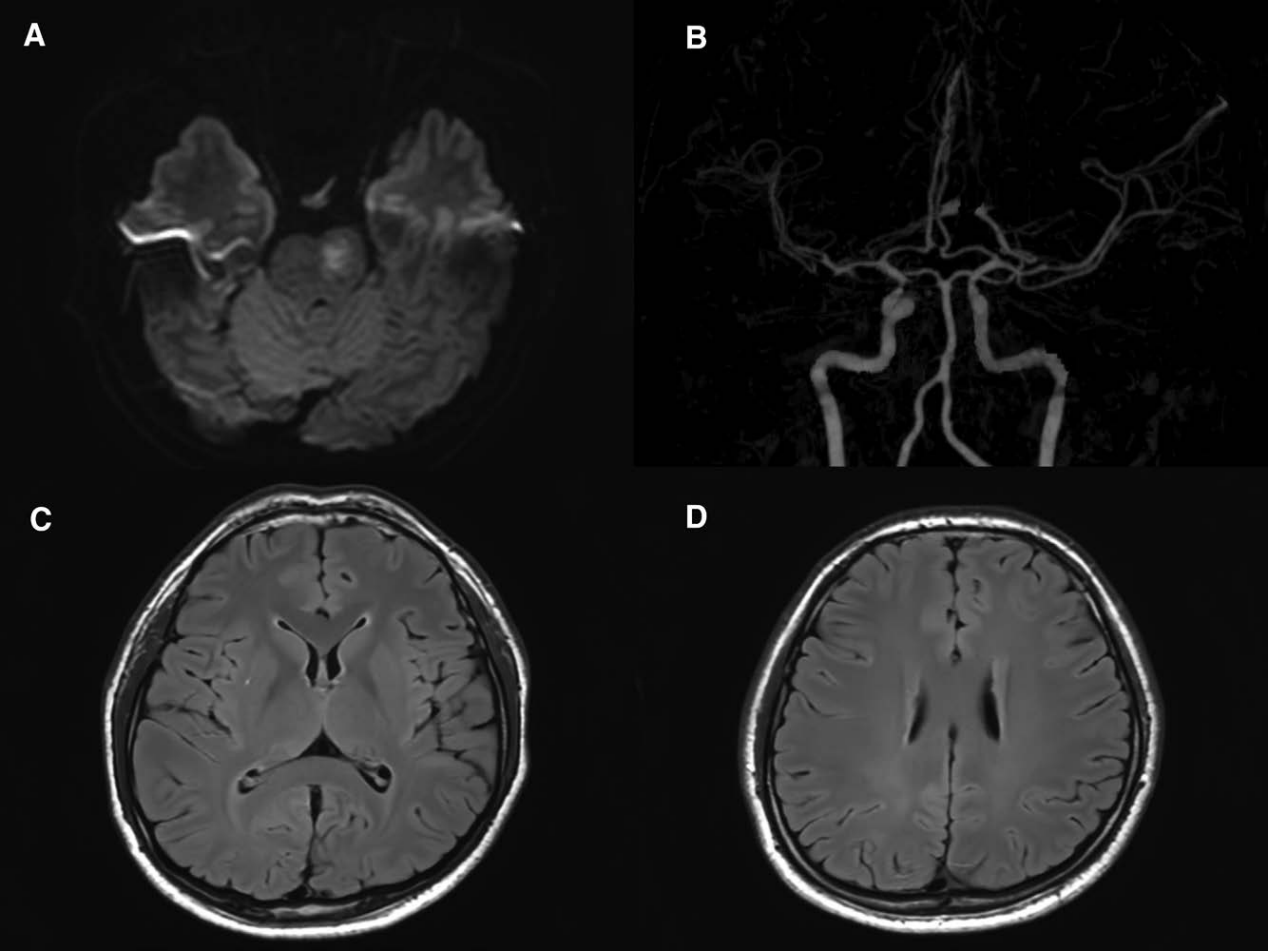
(A) Brain magnetic resonance image (MRI) reveals a diffusion restriction lesion in the left pons. (B) Brain computed tomography angiography shows no significant stenosis or occlusion in the major intracerebral arteries. (C and D) T2-fluid attenuated inversion recovery MRI reveals no other brain lesions in the basal ganglia, frontal cortex, and subcortex.
